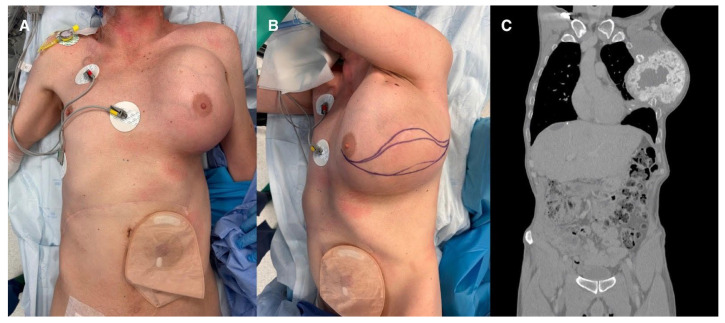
(A). Patient before surgery anterior view. (B). Patient before surgery lateral view. (C). Patient before surgery CT scan anterior view (This figure should be printed in colour).
